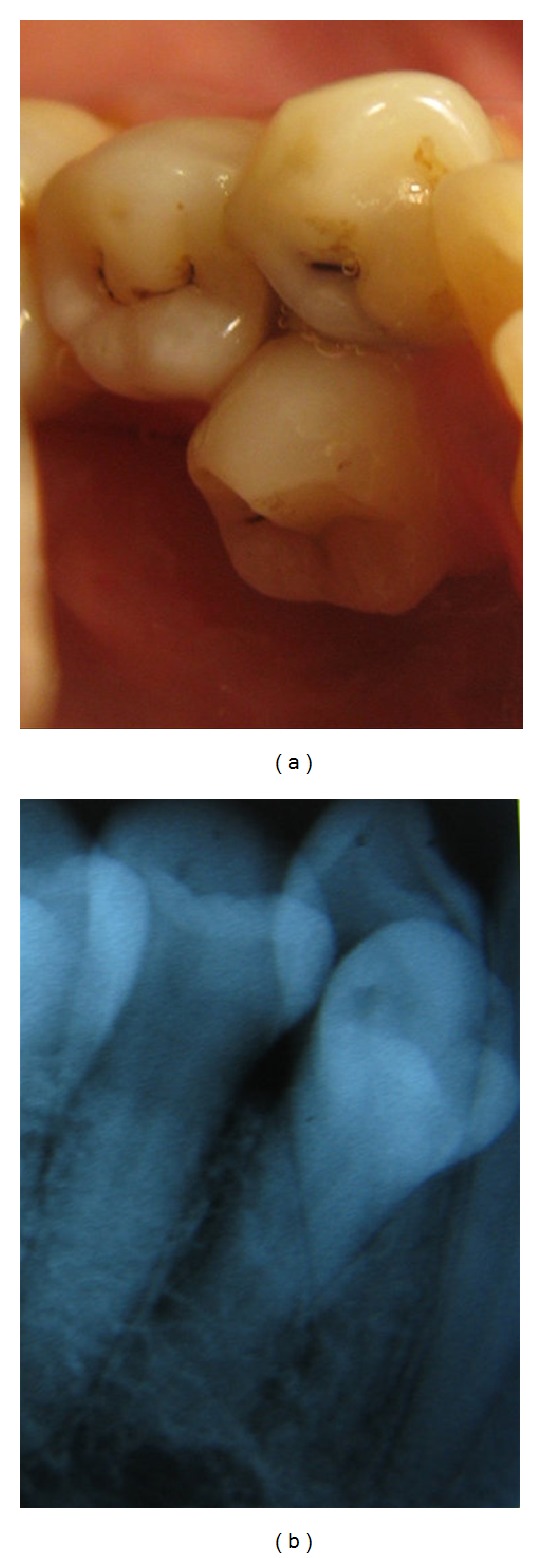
Clinical (a) and radiographic (b) images of a supernumerary fully formed lower bicuspid with an exaggerated linguoocclusal inclination that could add to the endodontic challenge of visibility, access, and instrumentation.
